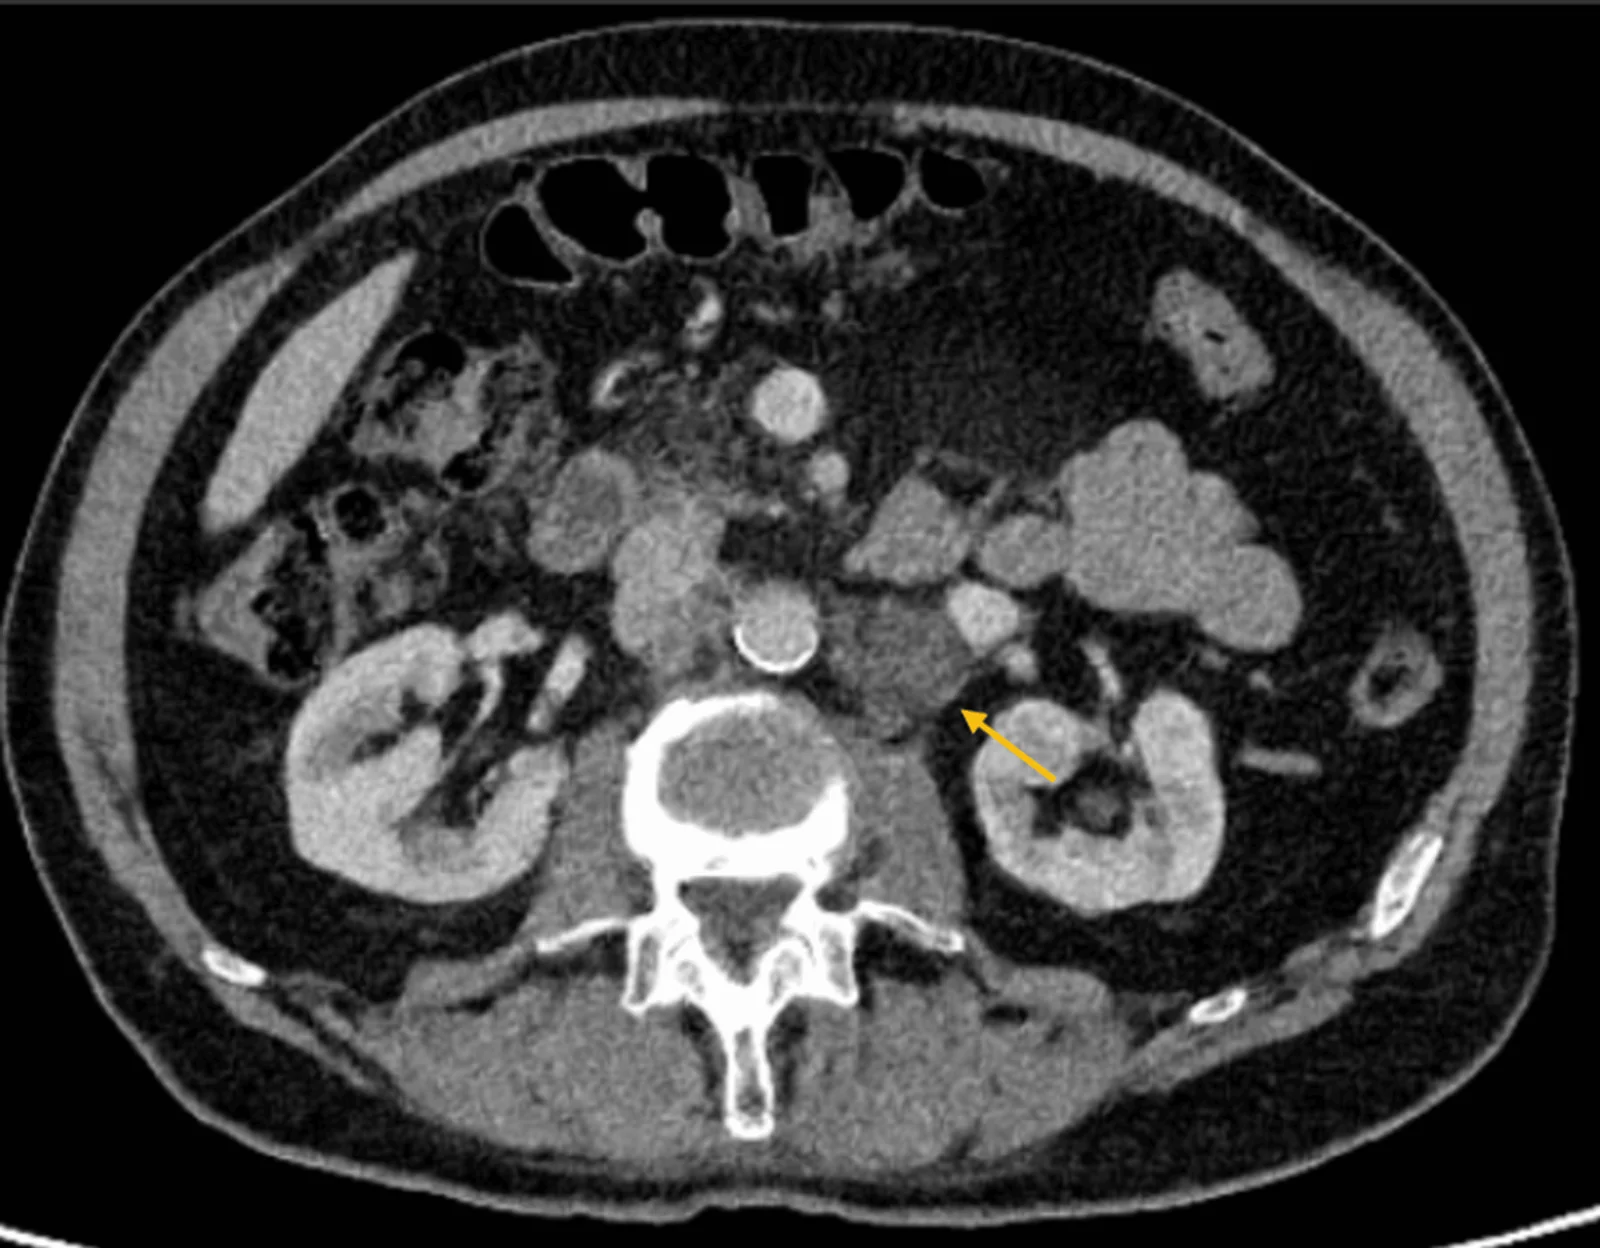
Contrast-enhanced axial CT image demonstrating isolated progression of the left para-aortic lymph node (yellow arrow), consistent with oligoprogression.
